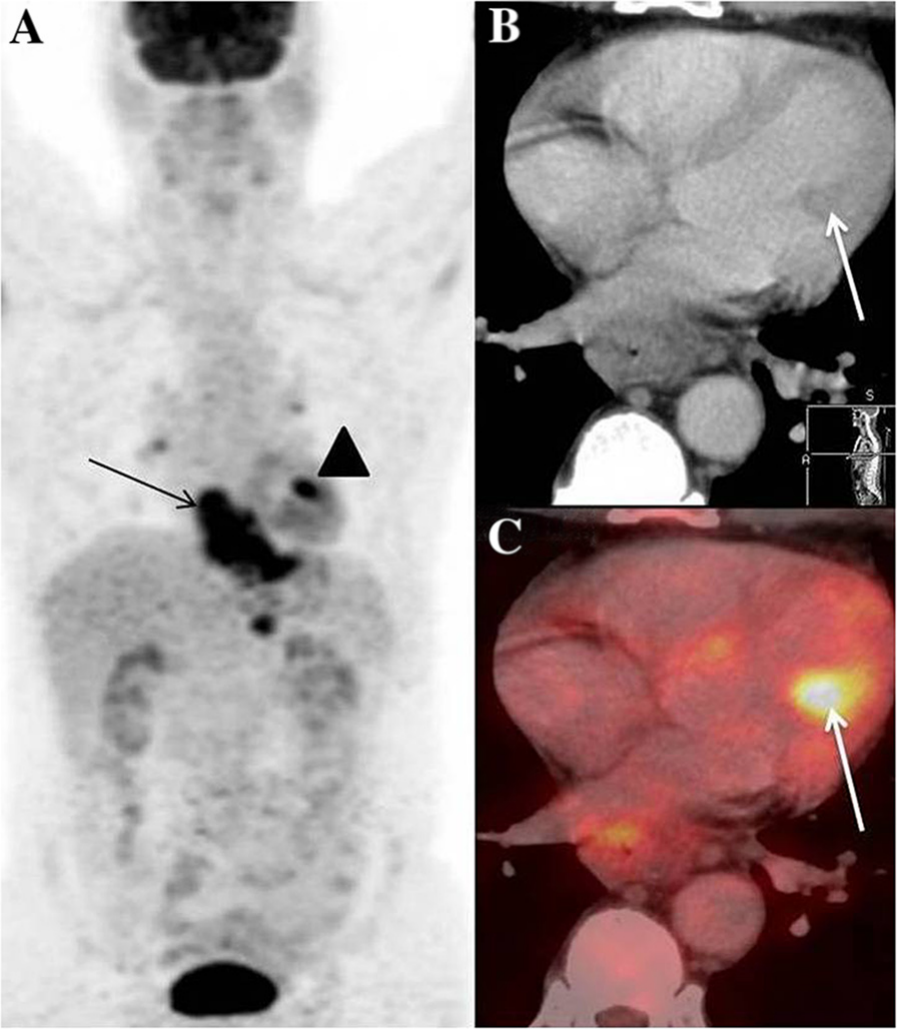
Myocardial metastases from esophageal cancer. A- MIP image showing primary esophageal mass (arrow) with focal uptake in region of heart (arrowhead), B- Hypodense lesion in left ventricular myocardium (arrow) on axial CT images showing, C- FDG uptake on axial fused PET/CT images (arrow).
